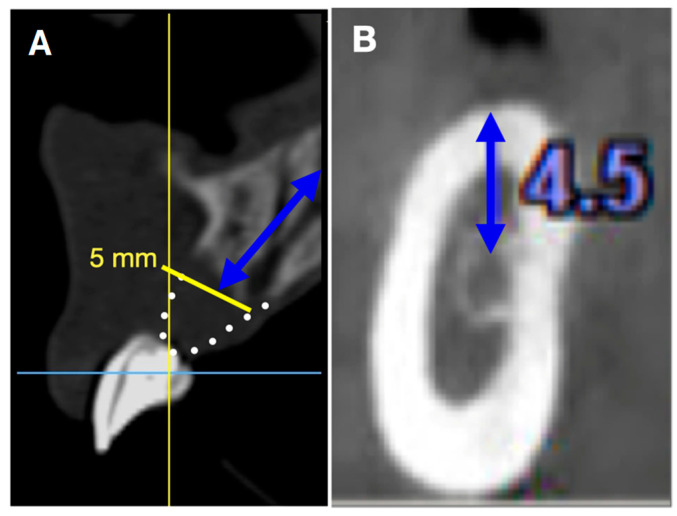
Cone-beam computed tomography (CBCT) reference methodology used for baseline vertical bone defect (VBD) measurements (T0). (A) Measurement of anterior maxillary vertical defect height, defined as the distance from the nasal floor to the viable edge of the residual alveolar crest. (B) Measurement of mandibular vertical defect height, defined as the distance from the viable edge of the residual alveolar crest to the superior border of the inferior alveolar nerve canal.
